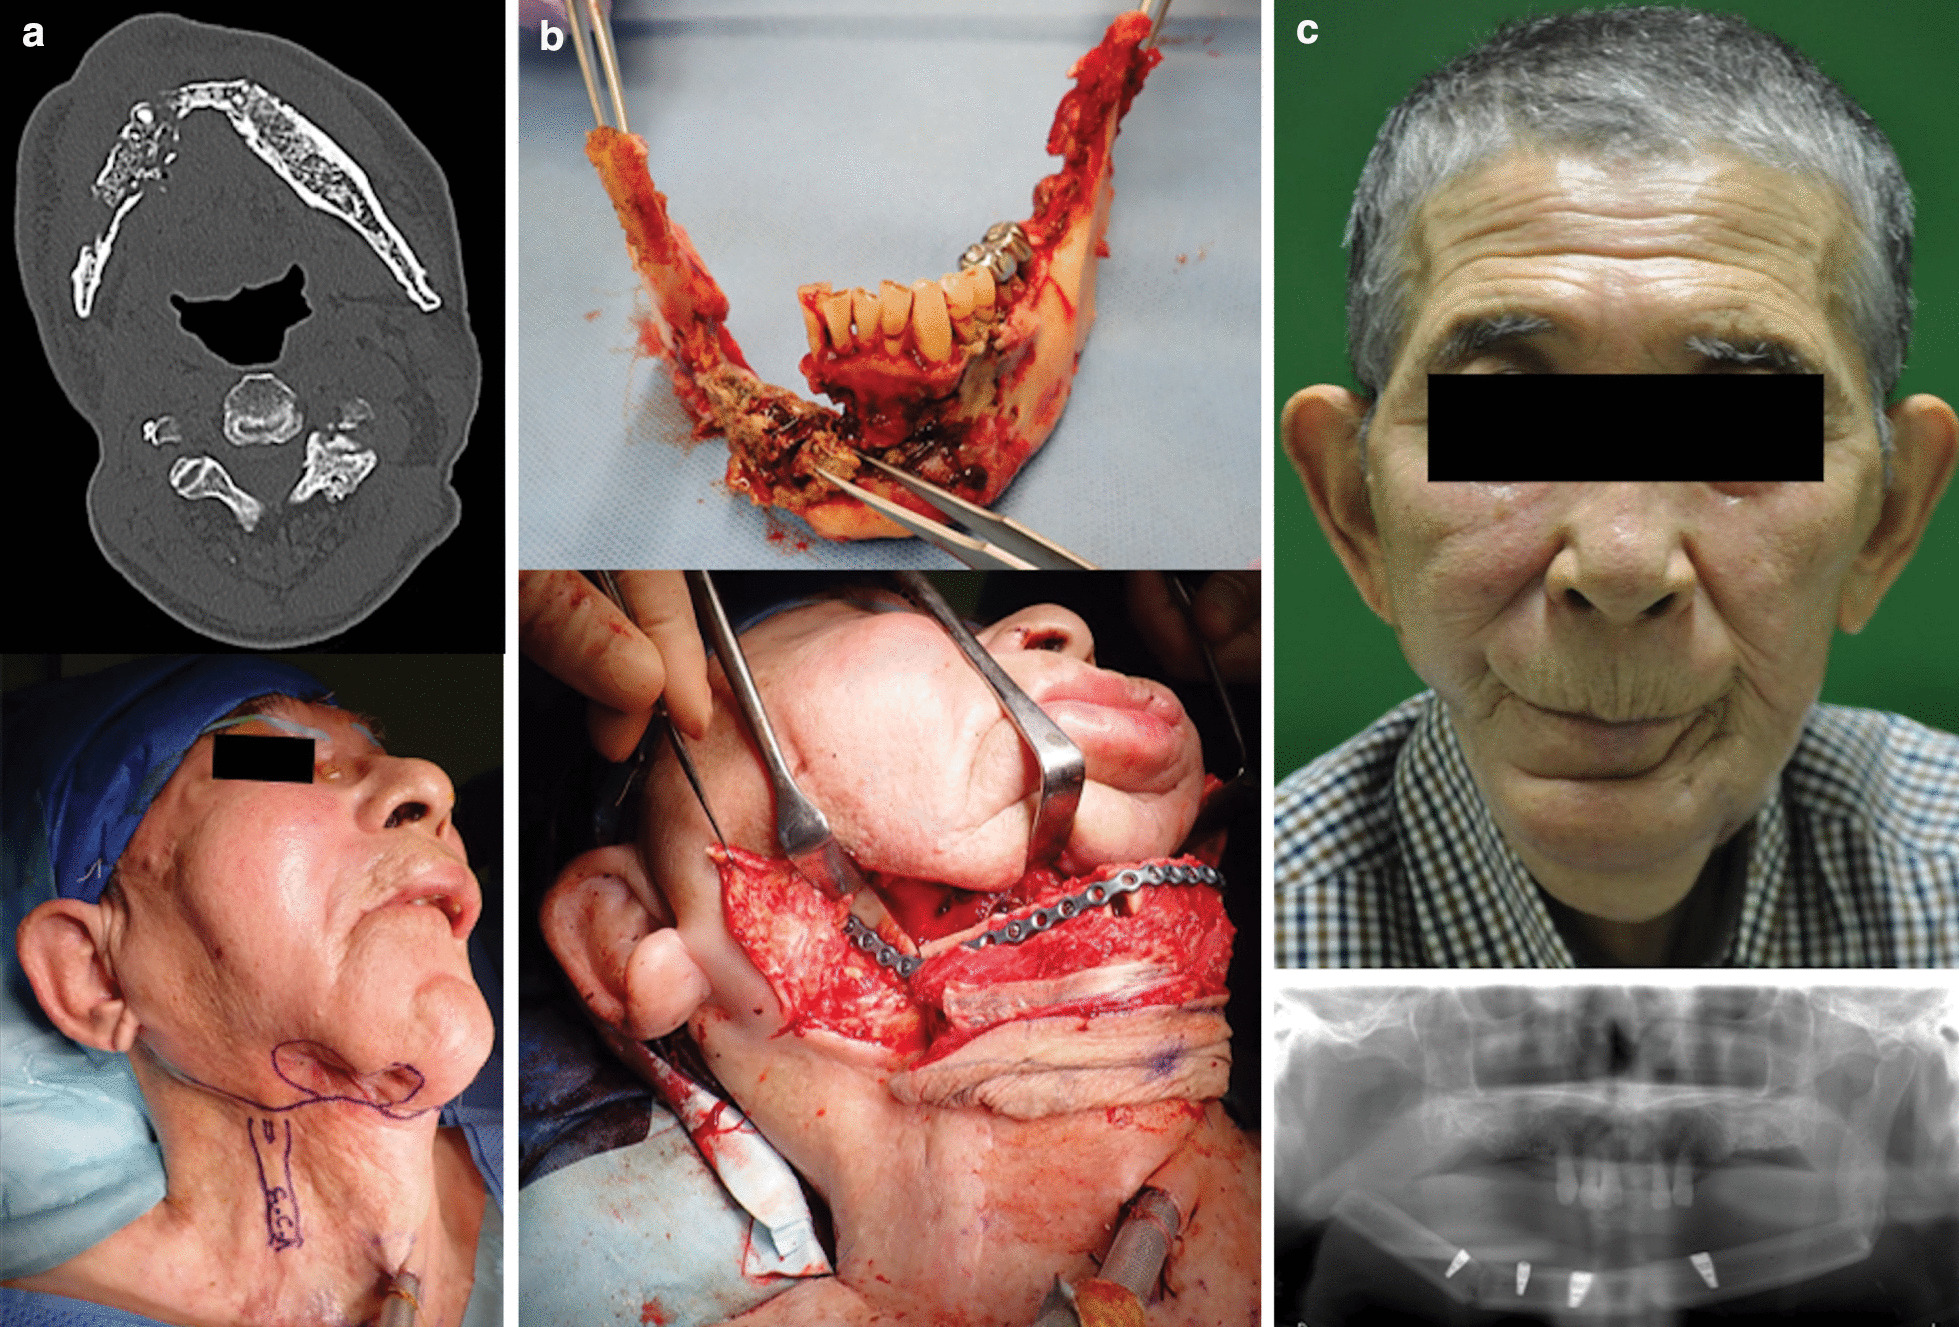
a Preoperative CT of patient 3 indicates subcutaneous abscesses and osteolysis (left, patient 3 in Table 1). Preoperative image shows an infected fistula discharging pus due to osteoradionecrosis (right). b The necrotized mandible was removed and healthy bilateral mandibular condyles were preserved (top). Osteotomy was performed in two places in the 19-cm-long fibula and the graft was transplanted into the mandibular defect (bottom). c Seven months after surgery, the flap survived completely and dental implant installation was successfully achieved. A scar revision around the mouth was proposed, however the patient did not provide consent for the same and was satisfied with the achieved results
